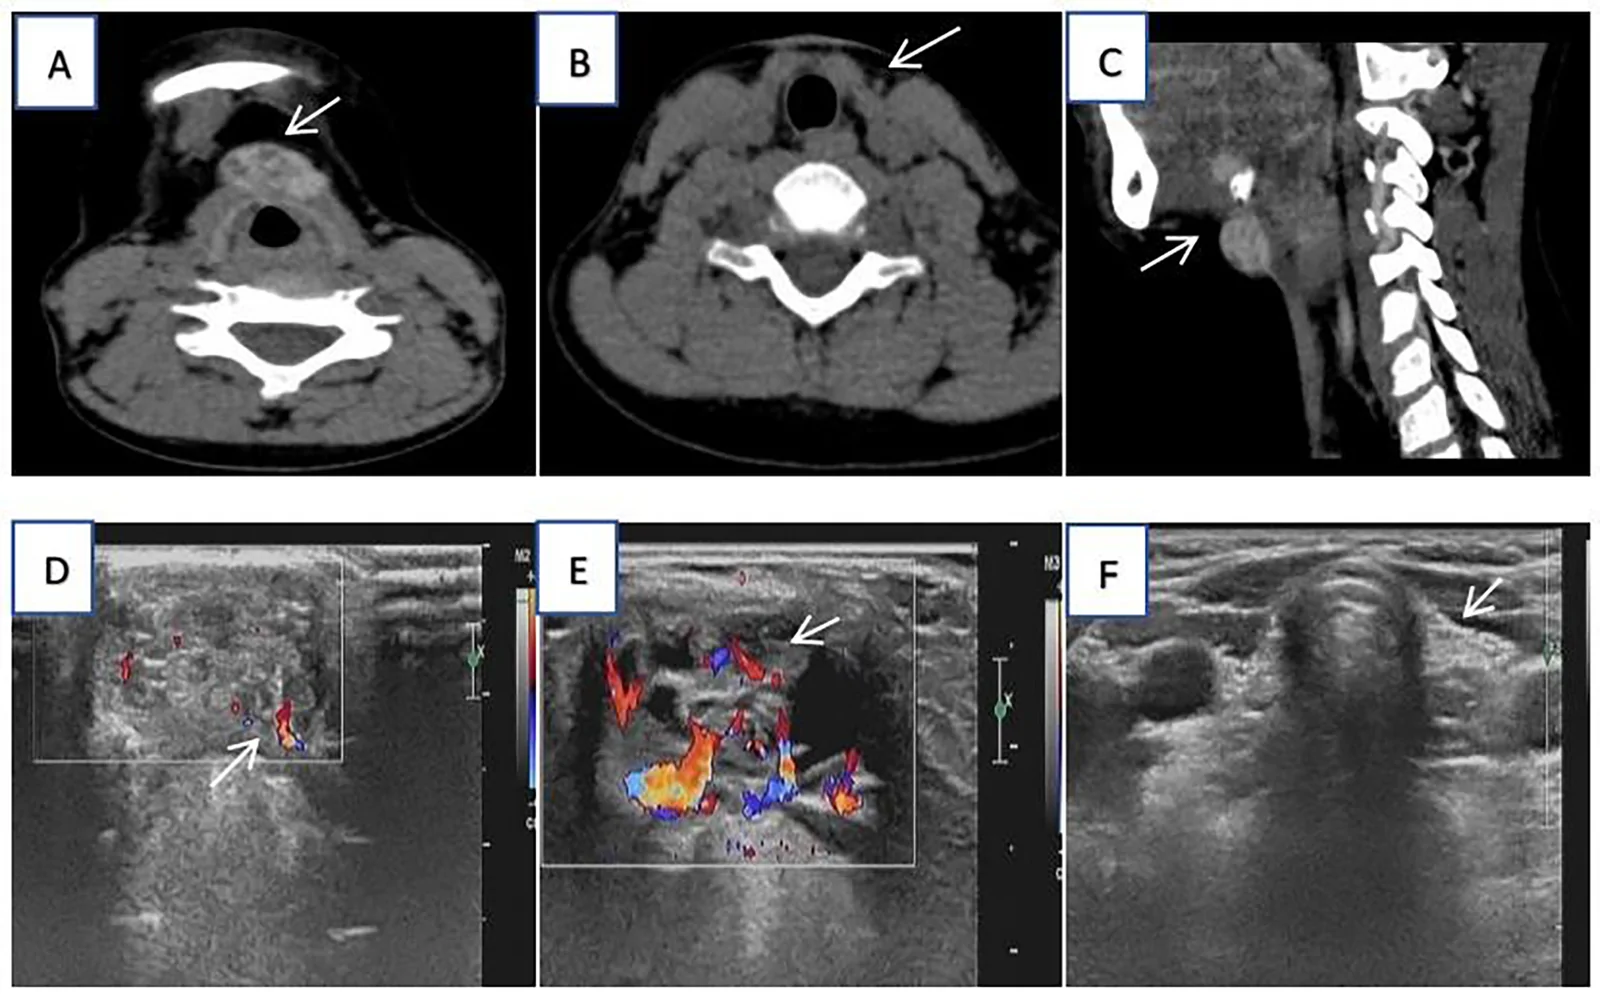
Imaging findings of case 2. (A) Ectopic thyroid tissue adjacent to the hyoid bone (white arrow). (B) No gland was visualized in the normal thyroid bed (white arrow). (C) Nodular ectopic thyroid tissue was observed above and below the hyoid bone. (D,E) Color Doppler ultrasonography showed blood-flow signals within the lesion (white arrows), supporting the diagnosis of ectopic thyroid. (F) No thyroid tissue was visualized in the normal paratracheal thyroid bed. The absence of eutopic thyroid tissue, together with hypothyroidism and vascularized tongue-base nodules, supported the diagnosis of lingual ectopic thyroid without normally located thyroid tissue.
